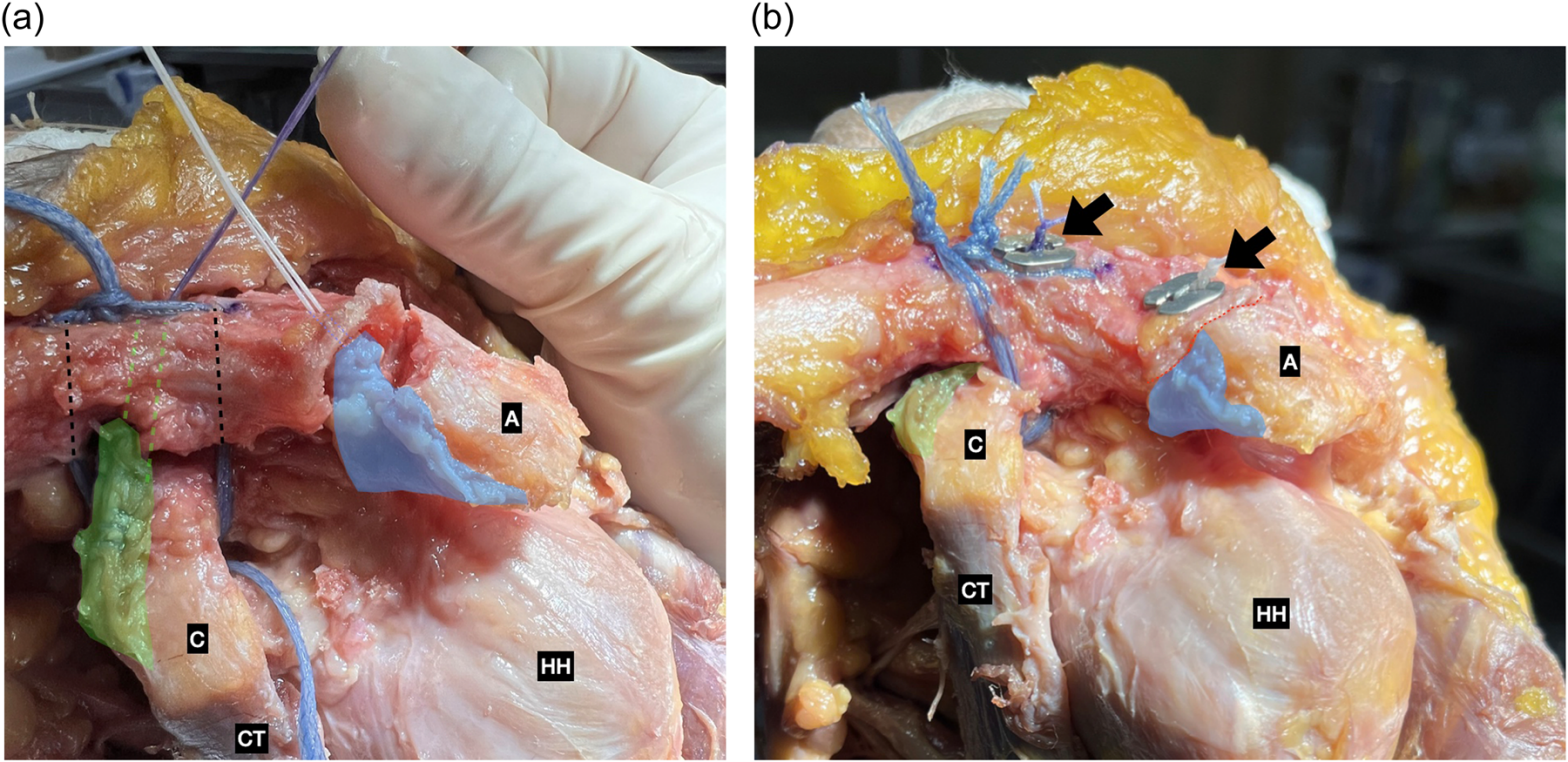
(a) Anterior view of a left shoulder. Biplanar reconstruction with double ultrahigh‐strength suture cerclage. The pectoralis minor tendon graft (highlighted in green) passed through the clavicle in an independent 4.0 mm tunnel (green dotted line) and two 2.5 mm tunnels for double fibertape fixation (black dotted lines) and CAL graft (highlighted in blue) transfer through the lateral clavicle resection. (b) Anterior view of a left shoulder. Biplanar reconstruction with double ultrahigh‐strength suture cerclage. Final construct with the pectoralis minor tendon (highlighted in green) associated with two double fibertape fixation and CAL transfer (highlighted in blue) through lateral clavicle resection. Both grafts are fixed with a superior cortical bottom (black arrows). A, acromion; C, coracoid; CAL, coracoacromial ligament; CT, conjoined tendon; HH, humeral head.
